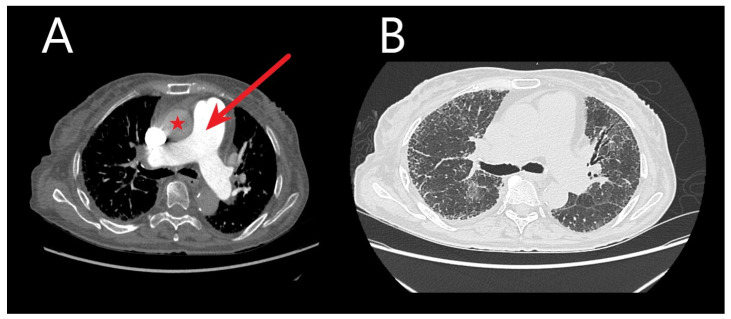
Chest computed tomography (CT) images without and with contrast are shown from an idiopathic pulmonary fibrosis patient. (A) Transaxial images are shown demonstrating an enlarged main pulmonary artery (red arrow) compared to the ascending aorta (red star) at the same level—suggestive of pulmonary hypertension. (B) Transaxial images in the lung window demonstrate bilateral honeycombing, intralobular, and interstitial thickening.
